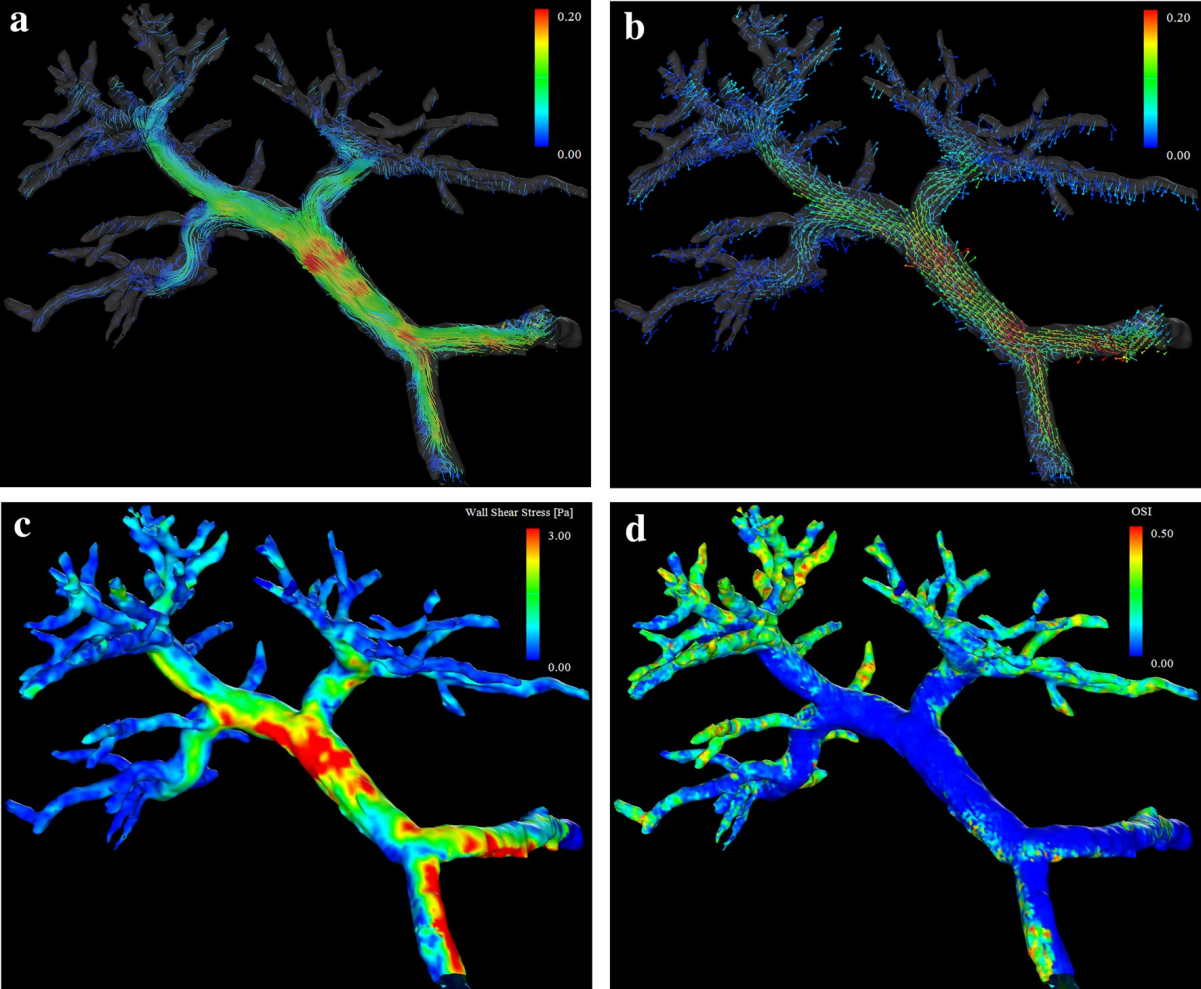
4D Flow MRI acquires the flow velocity vector data all at once, allowing for various types of post-analysis. Same case as Fig. 1. a A streamline is lined connecting tandem velocity vectors. b A 3D vector field is an assignment of a vector to each point in a subset of space. c The wall shear stress (WSS) is defined as the product of the fluid viscosity and the shear rate near the vessel wall. d The oscillatory shear index reflects the temporal variation in WSS and is calculated using the temporal variation in the local WSS vector. *OSI*, oscillatory shear index
